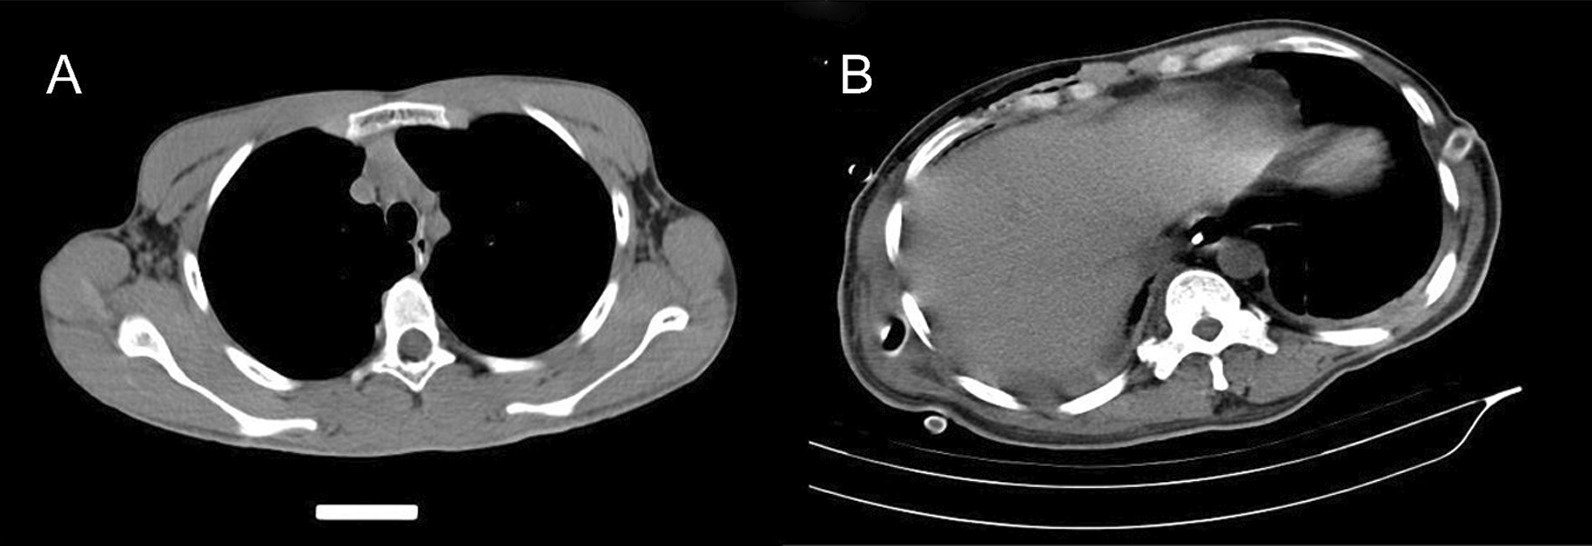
Computed tomographic images. A Esophageal perforation caused by foreign body. B Esophageal fistula
